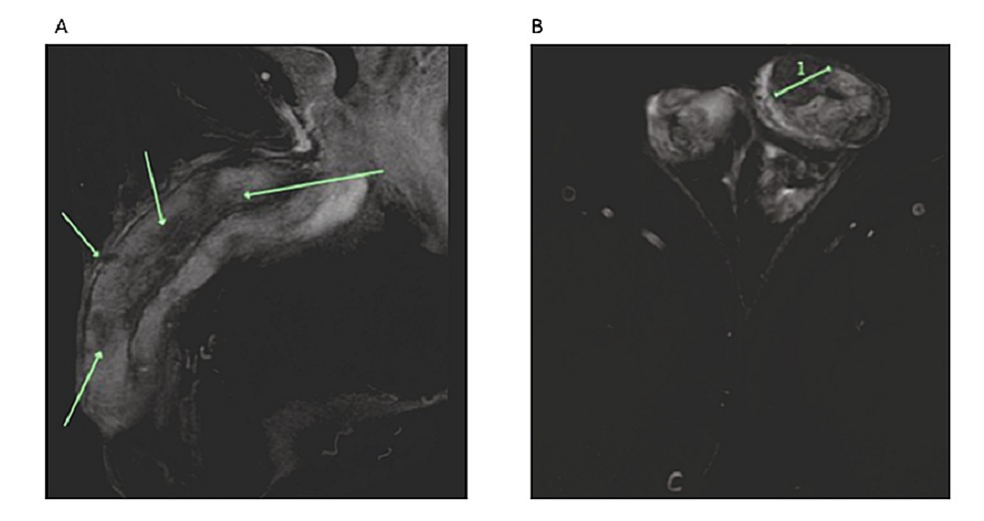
Penile MRI scan of the patient A) Sagittal view of T1 fat suppression sequence with contrast. Arrow: reduced contrast uptake. B) Axial view of T2 fat suppression sequence, 1 Length: 1.92 cm
